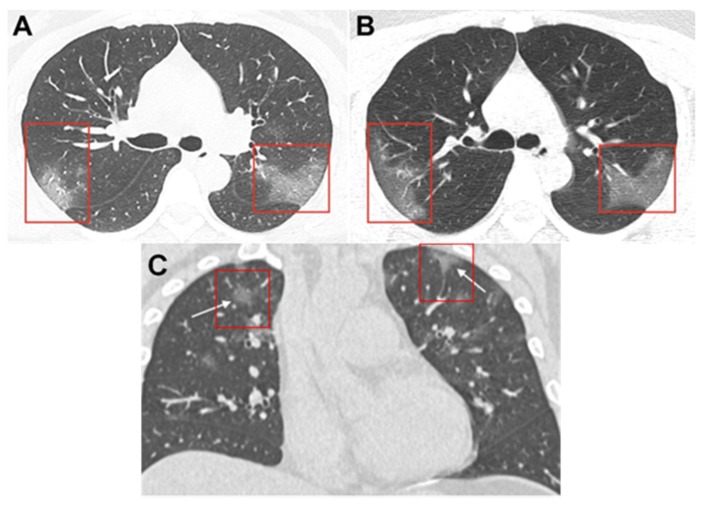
Unenhanced computerized tomography (CT) images of infected lungs of a 33-year-old woman. (A) Image showing multiple ground-glass opacities in bilateral lungs [33]; (B) image obtained 3 days after follow-up showing progressive ground-glass opacities in the posterior segment of the right upper lobe and apical posterior segment of left superior lobe [33]; (C) coronal thin-section non-contrast CT image of infected lungs of a 36-year-old male showing ground-glass opacities with a rounded morphology in both upper lobes [34].
